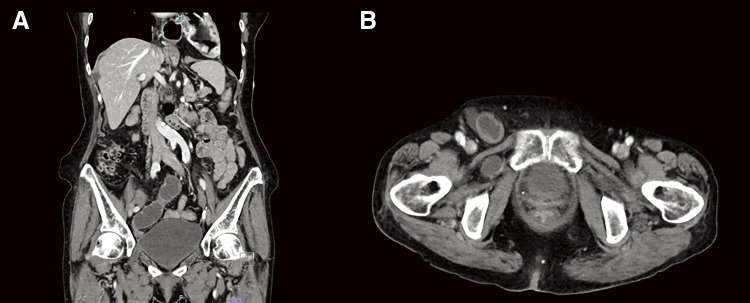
Contrast-enhanced abdominal CT scan (A: coronal section; B: axial section). A right femoral hernia was found. There was no area of poor contrast in the mated intestine. The bladder was located in the right obturator foramen. CT, computed tomography
